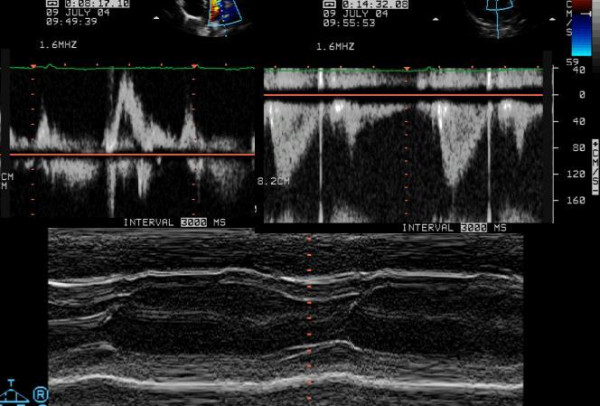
Normal echocardiogram without left ventricular hypertrophy.
